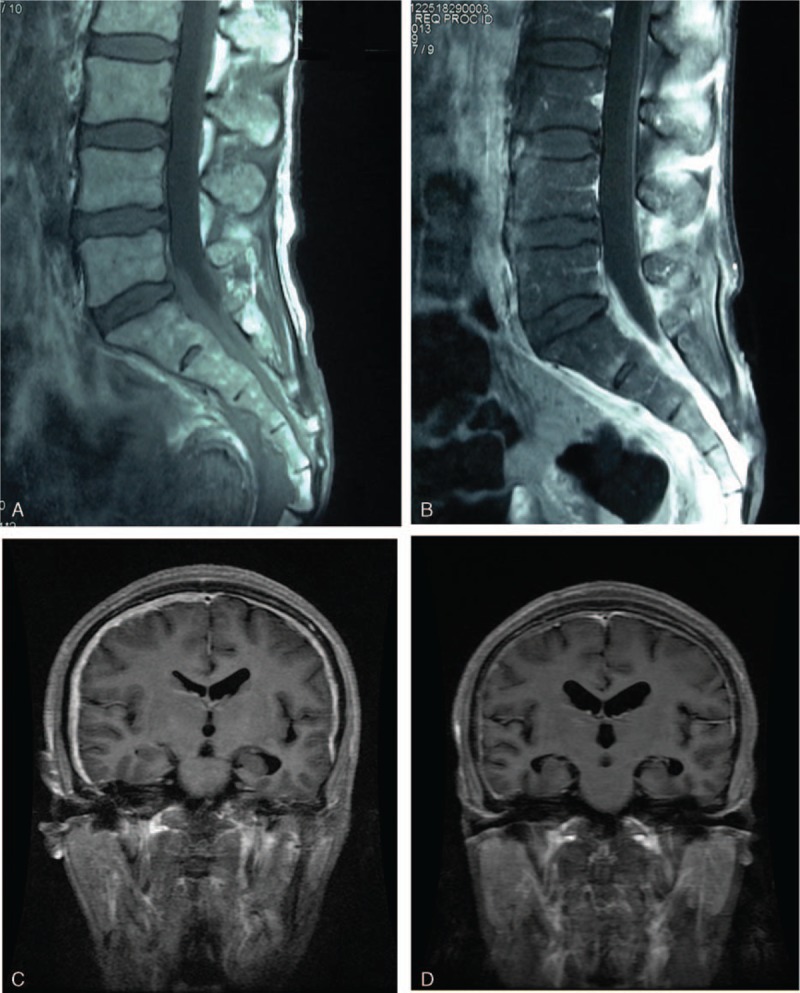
Lumbar magnetic resonance imaging (MRI) showed dural thickening (A) with homogenous gadolinium enhancement (B). Brain MRI revealed diffuse pachymeningeal enhancement over both cerebral convexities (C). Dural thickening greatly resolved 1 year after treatment with steroids and immunosuppressant (D).
